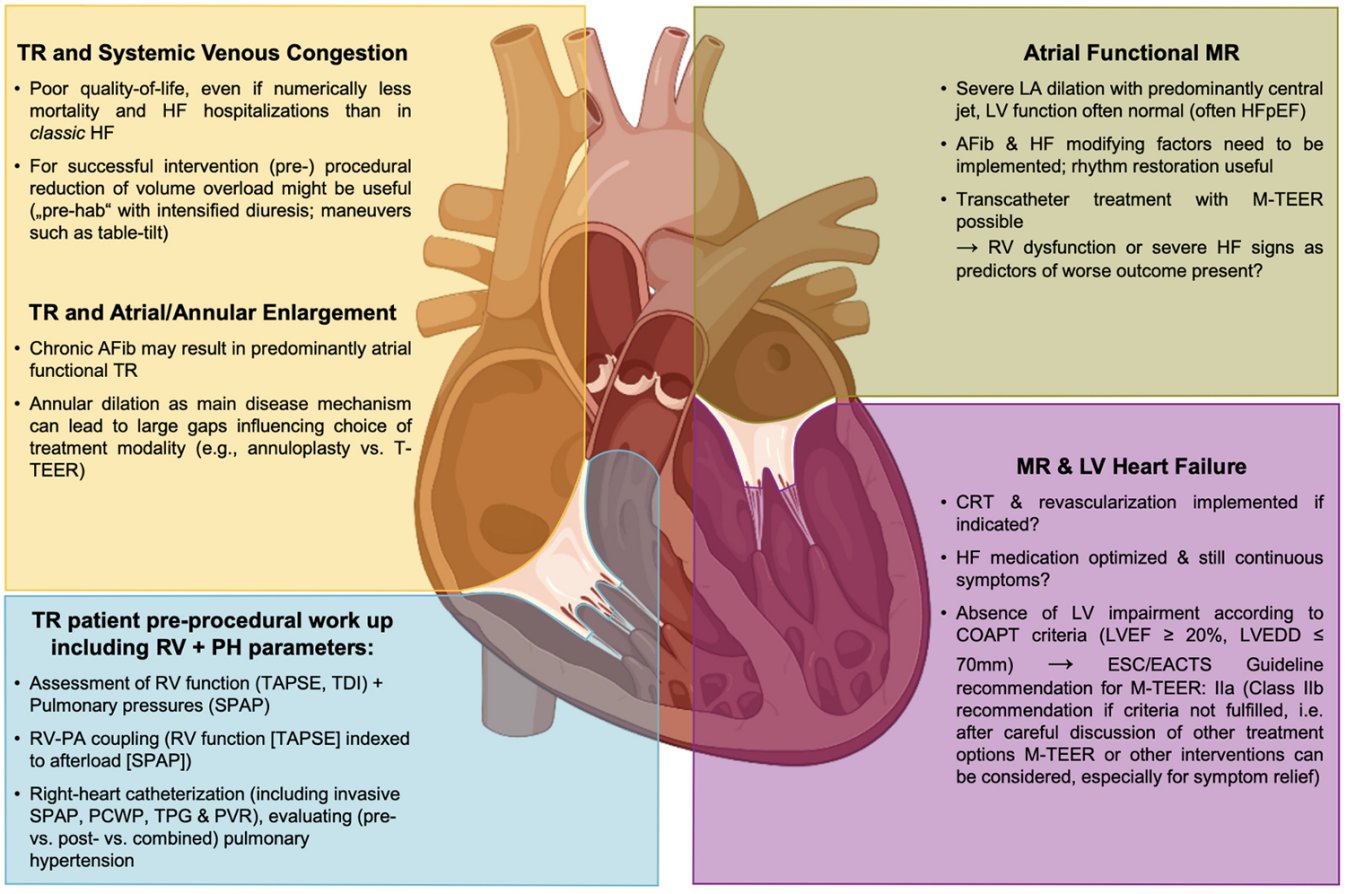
MR and TR in the HF disease conundrum and as part of a systemic disease—implications for interventional therapies. AFib, atrial fibrillation; CRT, cardiac resynchronization therapy; HF, heart failure; HFpEF, heart failure with preserved ejection fraction; LA, left atrium; LV, left ventricle; LVEDD, left ventricular end-diastolic diameter; LVEF, left ventricular ejection fraction; MR, mitral regurgitation; M-TEER, mitral transcatheter edge-to-edge repair; PA, pulmonary artery; PCWP, pulmonary capillary wedge pressure; PVR, pulmonary vascular resistance; RV, right ventricle; SPAP, systolic pulmonary artery pressure; TAPSE, tricuspid annular plane systolic excursion, TDI, tissue Doppler imaging; TPG, trans pulmonary gradient; T-TEER, tricuspid transcatheter edge-to-edge repair, TR, tricuspid regurgitation.
